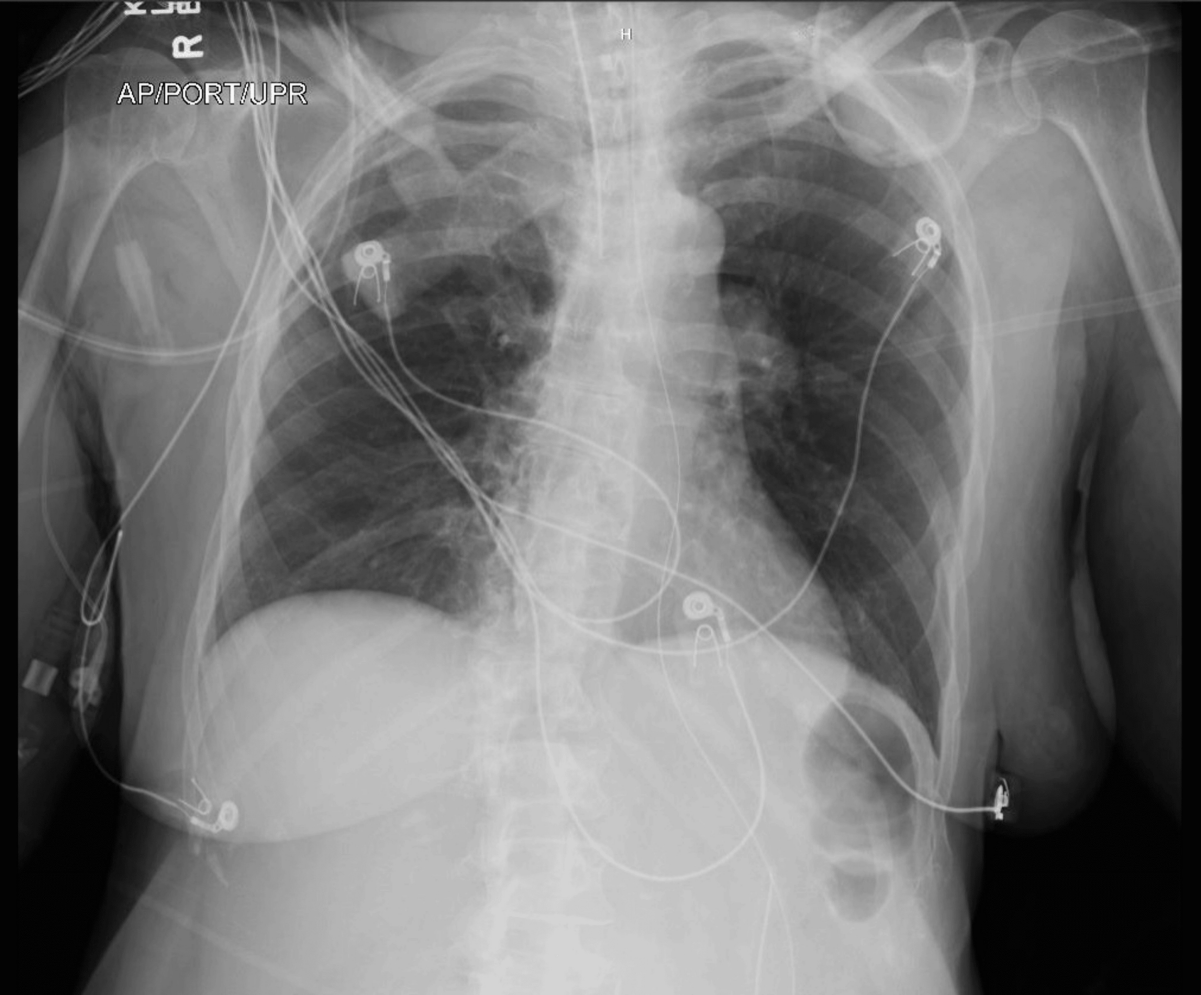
Portable upright anteroposterior chest X-ray showing no cardiopulmonary abnormalities.
